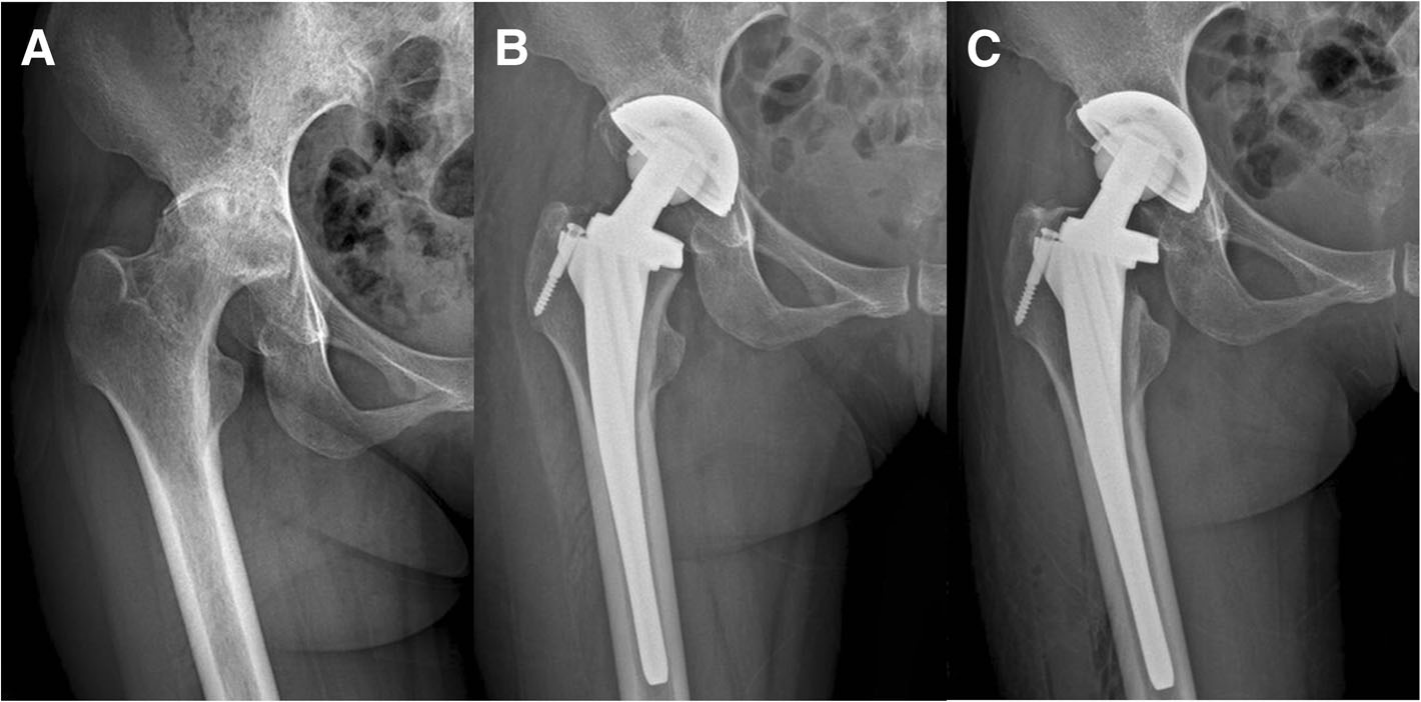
Preoperative anteroposterior radiograph of a 36-year-old woman showing femoral head osteonecrosis of the right hip (a). Anteroposterior radiograph 3 weeks after THA using a ribbed stem (b). Anteroposterior radiograph at 2-year follow-up showing osteolysis in Gruen zone 7 (c)
